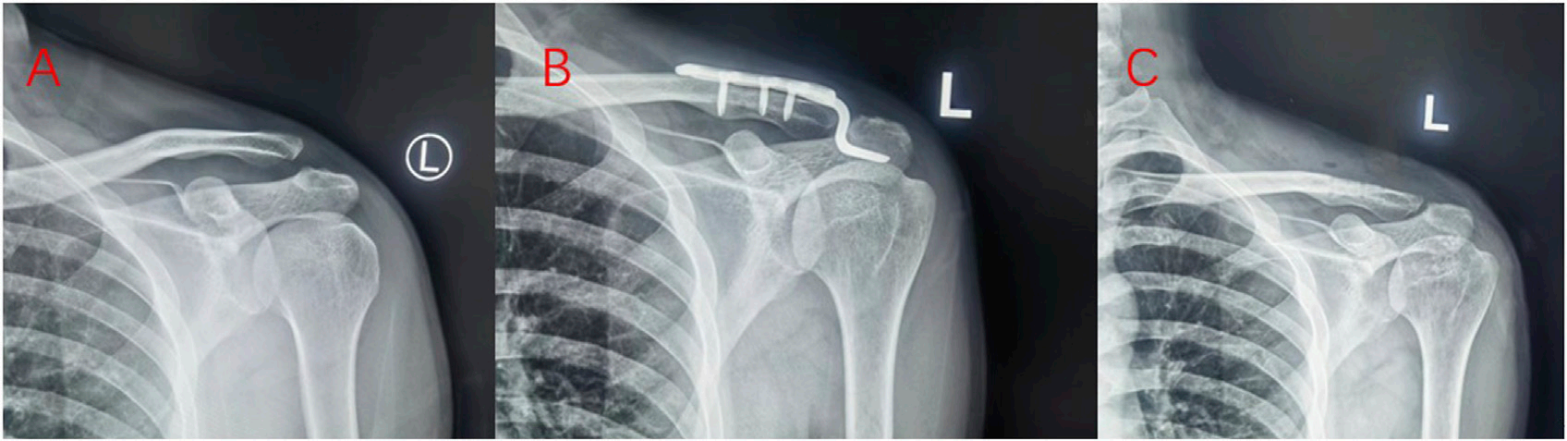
The acromioclavicular joint dislocation was fixed with the hook plate. Preoperative X-ray (A), X-ray on the second day after operation (B), and removal of hook plate X-ray (C) 8 months after operation.
